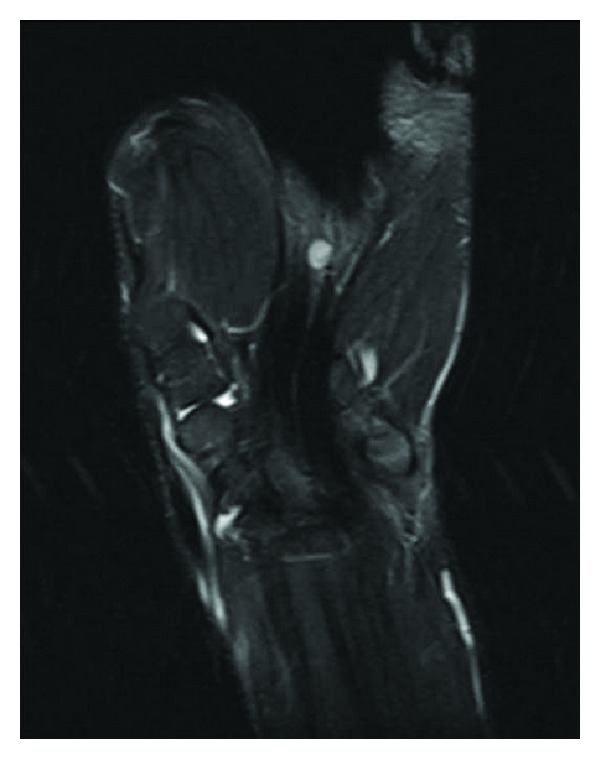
6 mm diameter mass in the palm which has intermediate signal on T1-weighted images and hyperintense signal on T2-fat weighted images was detected.
